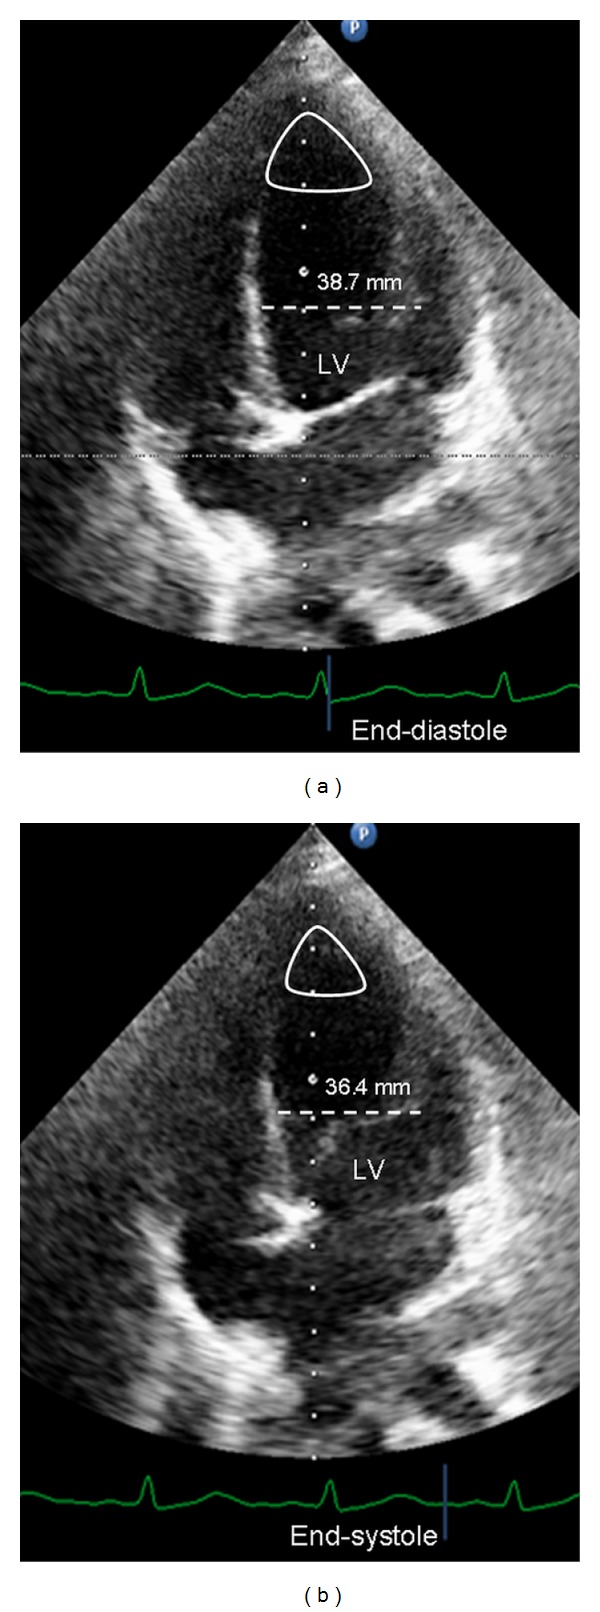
Transthoracic echocardiogram (4-chamber view) during end-diastole (a) and end-systole (b) shows basal and midventricular segmental hypokinesis (dotted line for reduced shortening) of the left ventricle (LV), with a well-preserved apical segmental systolic function (loop).
